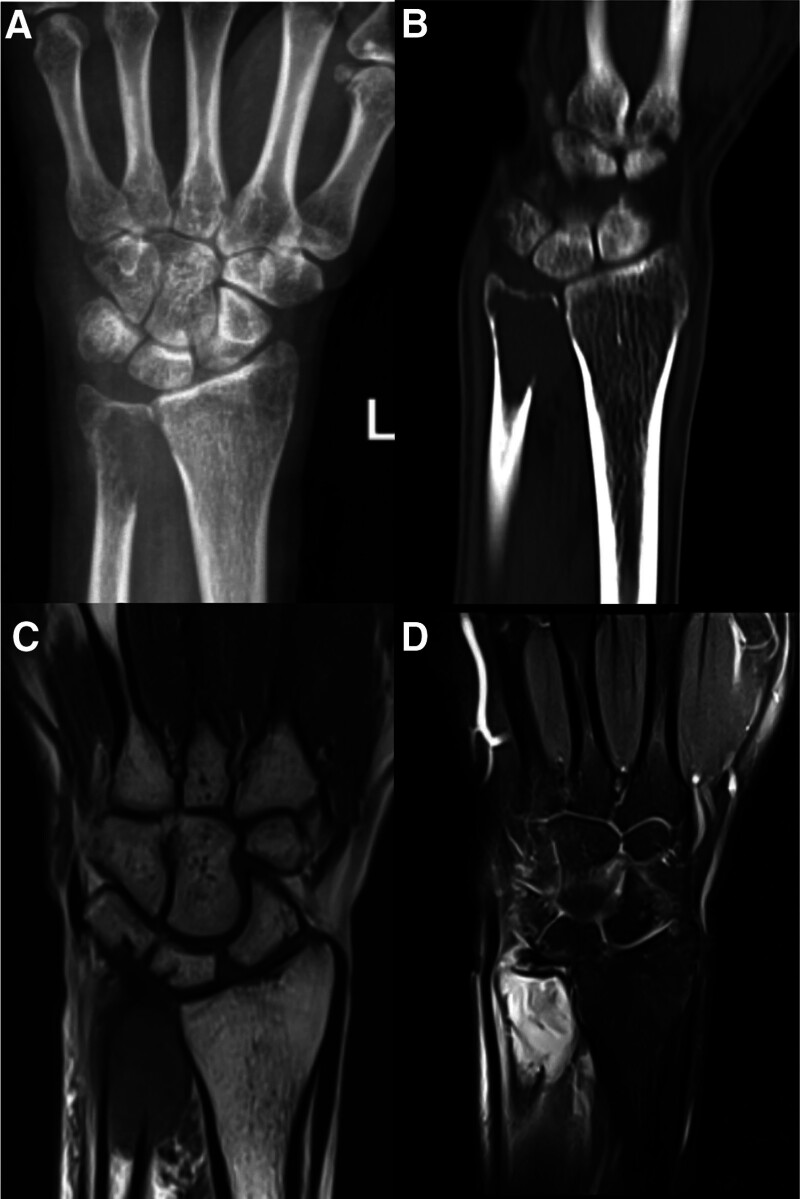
(A) X-ray demonstrated an expansile lytic lesion of the left distal ulna; (B) CT scans demonstrated the lesion has penetrated the cortex; (C) MRI of lesion in left wrist in T1-weighted image; and (D) T2-weighted image view.
